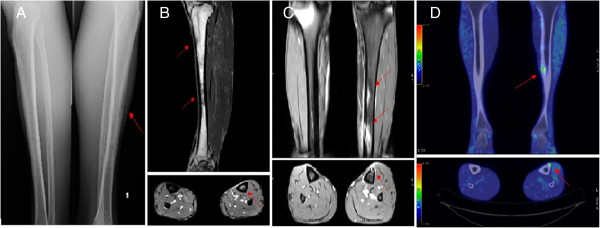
Morphological and functional imaging of the patient's bone lesions. A: Anterior-posterior x-ray image: focal bone loss of uncertain significance localized in the proximal and middle diaphysis of the left tibia. B: Sagittal and axial NMR images: focal alterations of the left tibia, both in the medullar and in the cortical portion, probably related to an inflammatory process. C: Coronal and axial NMR images: contrast-enhanced cortico-subcortical abnormalities in the middle-distal diaphysis of the left tibia. D: PET/CT fused images (coronal and axial): increased uptake of FDG in the diaphyseal cortex of the left tibia associated with structural alterations probably connected to inflammatory results.
